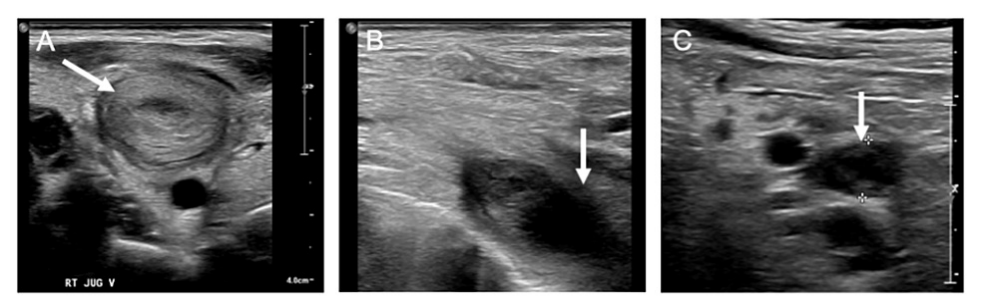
Duplex ultrasound of the upper extremity veins revealing diffuse deep vein thrombosis. Intraluminal echogenicity indicating thrombus (white arrow) in the right internal jugular vein (A), subclavian (B), and axillary (C).
